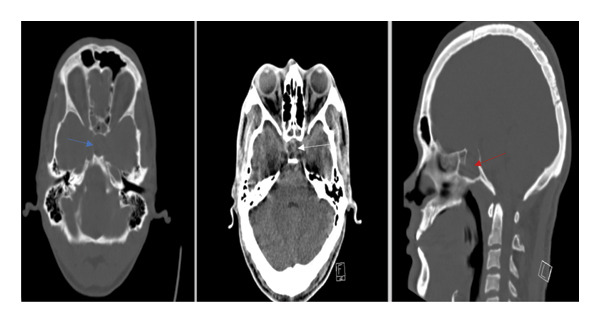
CT scan on admission. Selective nonenhanced CT scan images of the sellar region (axial bone window, axial brain window, and sagittal bone window) show a wide sella with thinning of the sella turcica bone (blue arrow). The sellar fossa is depressed (red arrow) and filled with a heterogeneous lesion without calcifications (white arrow).
